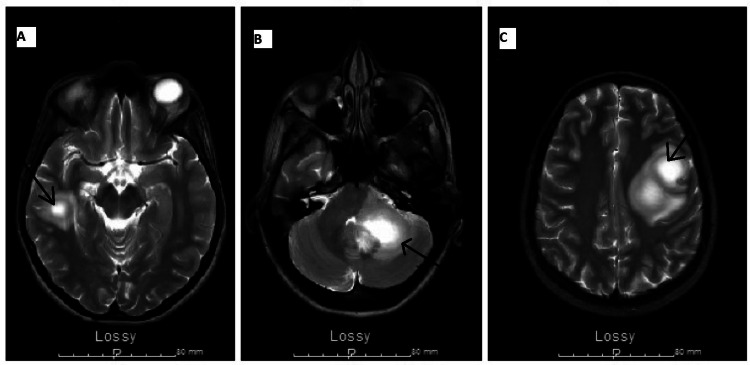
Transverse sections of T2-weighted brain magnetic resonance imaging showing enhancing lesions in the right cerebral (A), left cerebellar (B), and left cerebral hemispheres (C).
